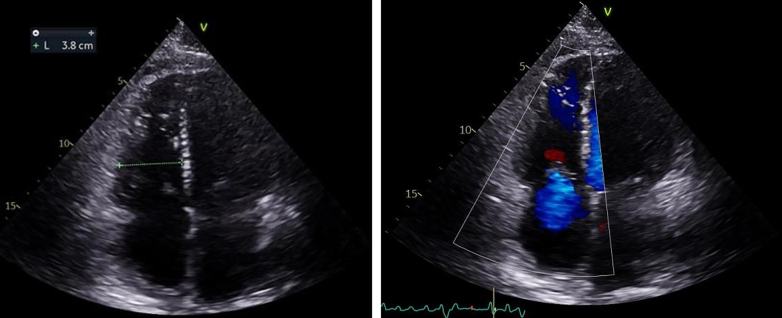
Follow-Up Echocardiography Follow-up echocardiography shows preserved left and right ventricular function, no mitral regurgitation, and moderate tricuspid regurgitation.
